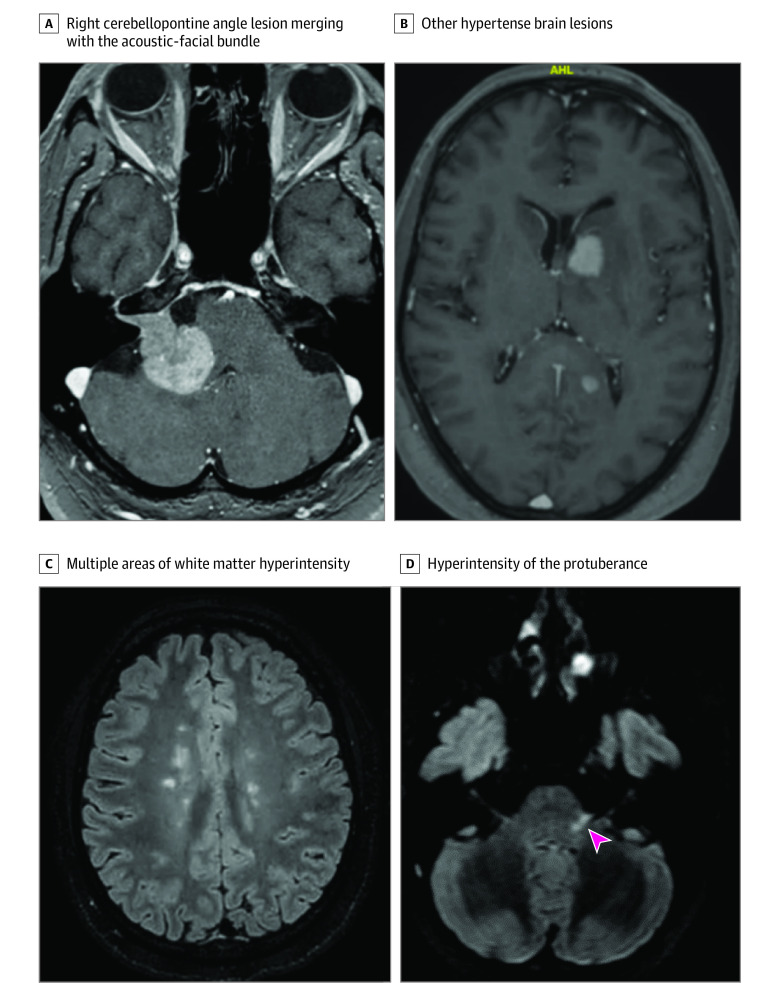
Magnetic Resonance Imaging of Patients With Peripheral Facial Palsy Due to Cerebral Lymphoma and Multiple Sclerosis A, Contrast-enhanced T1-weighted image in a patient in their 50s with right peripheral facial palsy (PFP) due to cerebral lymphoma showing right cerebellopontine angle lesion merging with the acoustic-facial bundle with enhancement after gadolinium injection. B, Contrast-enhanced T1-weighted image in the same patient showing other hypertense brain lesions. C, Multiple areas of white matter hyperintensity in a woman in her 20s with left PFP due to multiple sclerosis. D, Fluid-attenuated inversion recovery image in the same patient showing hyperintensity of the protuberance, seemingly in the root of the acoustic-facial bundle (arrowhead).
